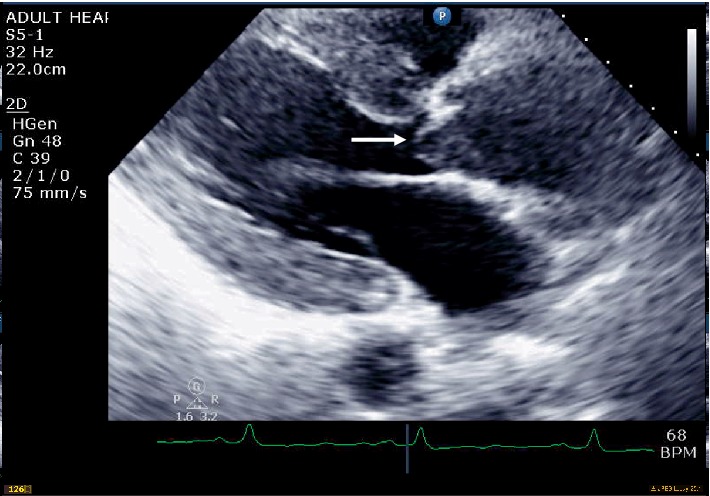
Parasternal long axis view showing dissection flap prolapsing (arrow) into the left ventricular outflow tract causing aortic insufficiency.
